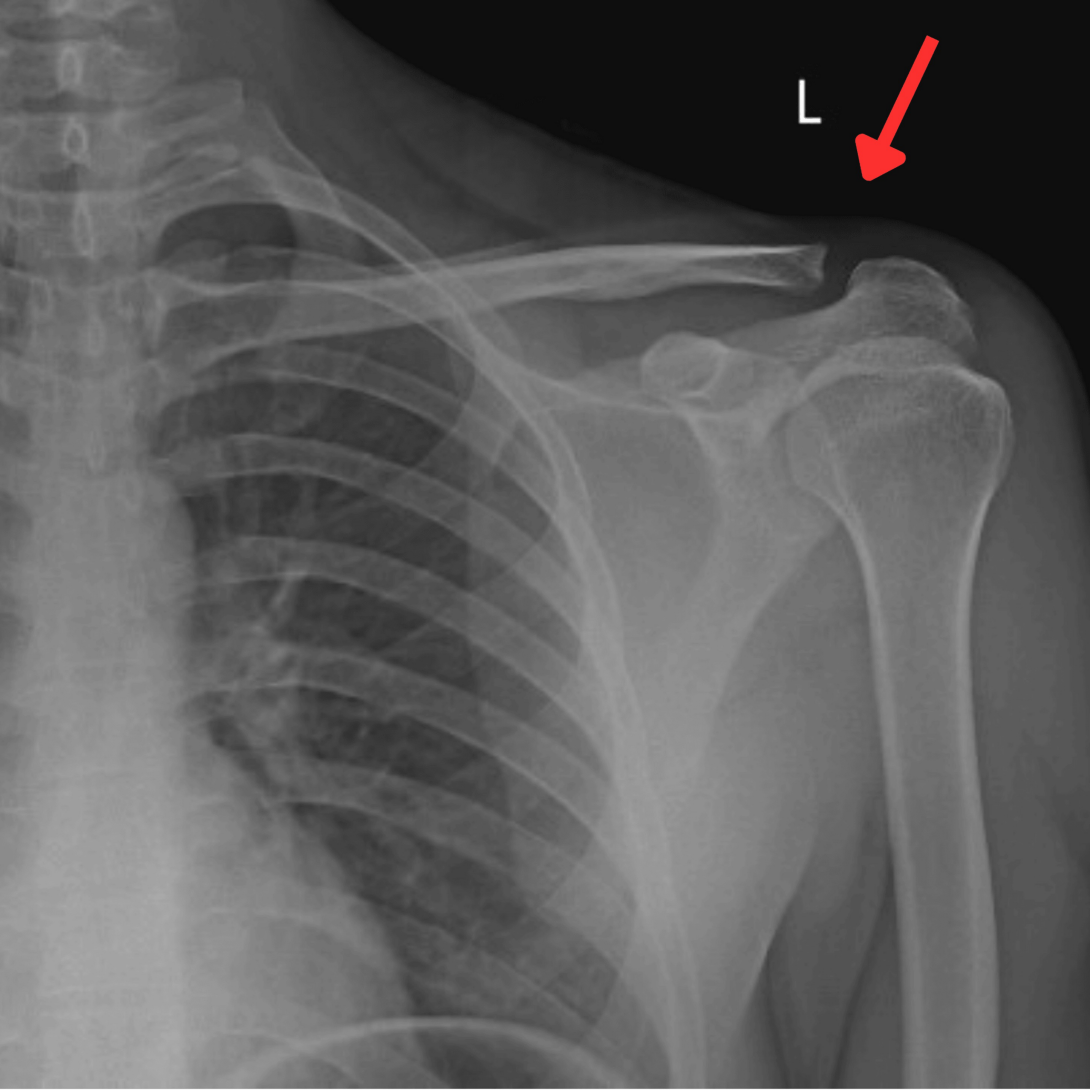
Radiograph of the left shoulder joint with grade II AC joint sprain AC: acromioclavicular
